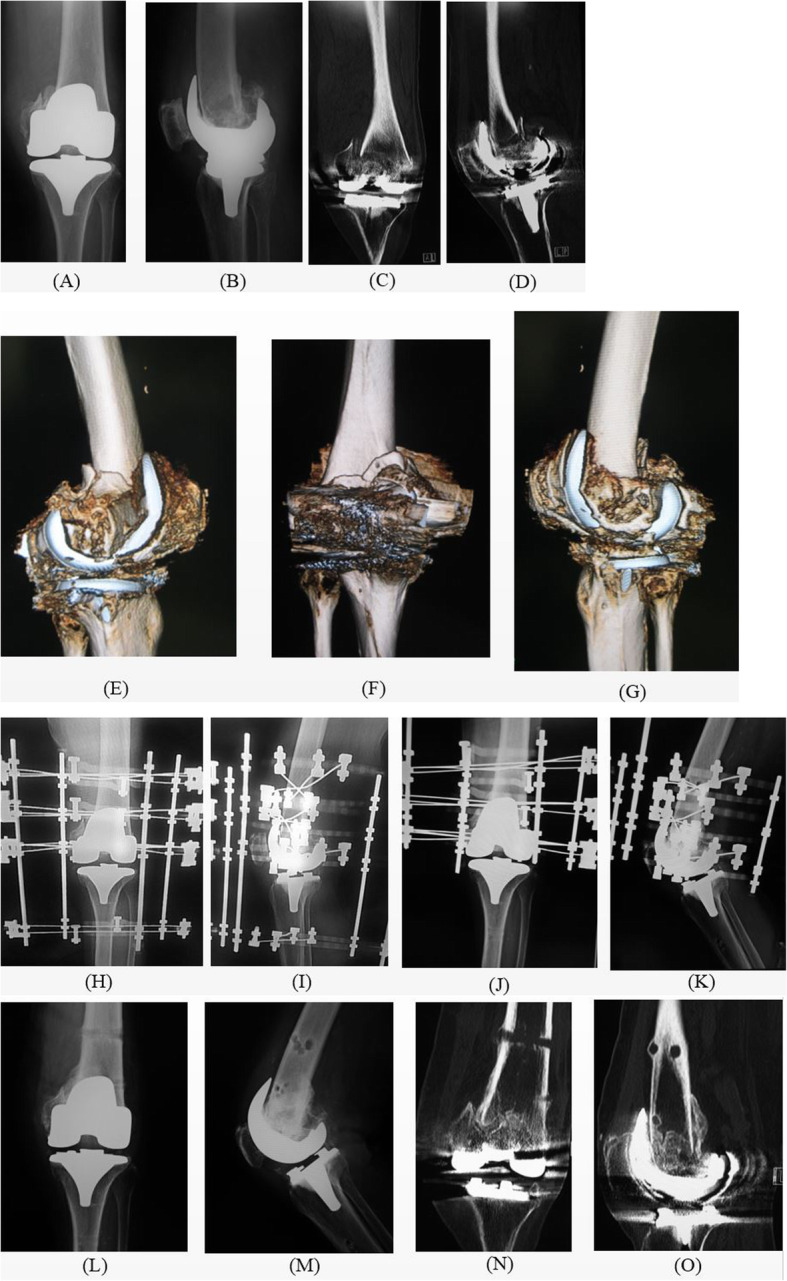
Periprosthetic distal femur fracture in case number 11 treated with a circular external fixator. (A) Preoperative antero-posterior X-ray. (B) Preoperative lateral angle X-ray. (C) Preoperative coronal CT scan. (D) Preoperative sagittal CT scan. (E) Preoperative medial-lateral angle 3D CT. (F) Preoperative posterior-anterior 3D CT. (G) Preoperative lateral-medial angle 3D CT. (H, I) Immediate postoperative X-ray. (J) Antero-posterior X-ray 2 weeks after removal of the tibial ring. (K) Lateral angle X-ray 2 weeks after removal of the tibial ring. (L,M) X-ray after circular external fixator removal and weight-bearing, postoperative 12 weeks. (N,O) CT after circular external fixator removal and weight-bearing, postoperative 12 weeks. CT, computed tomography; 3D, three-dimensional
